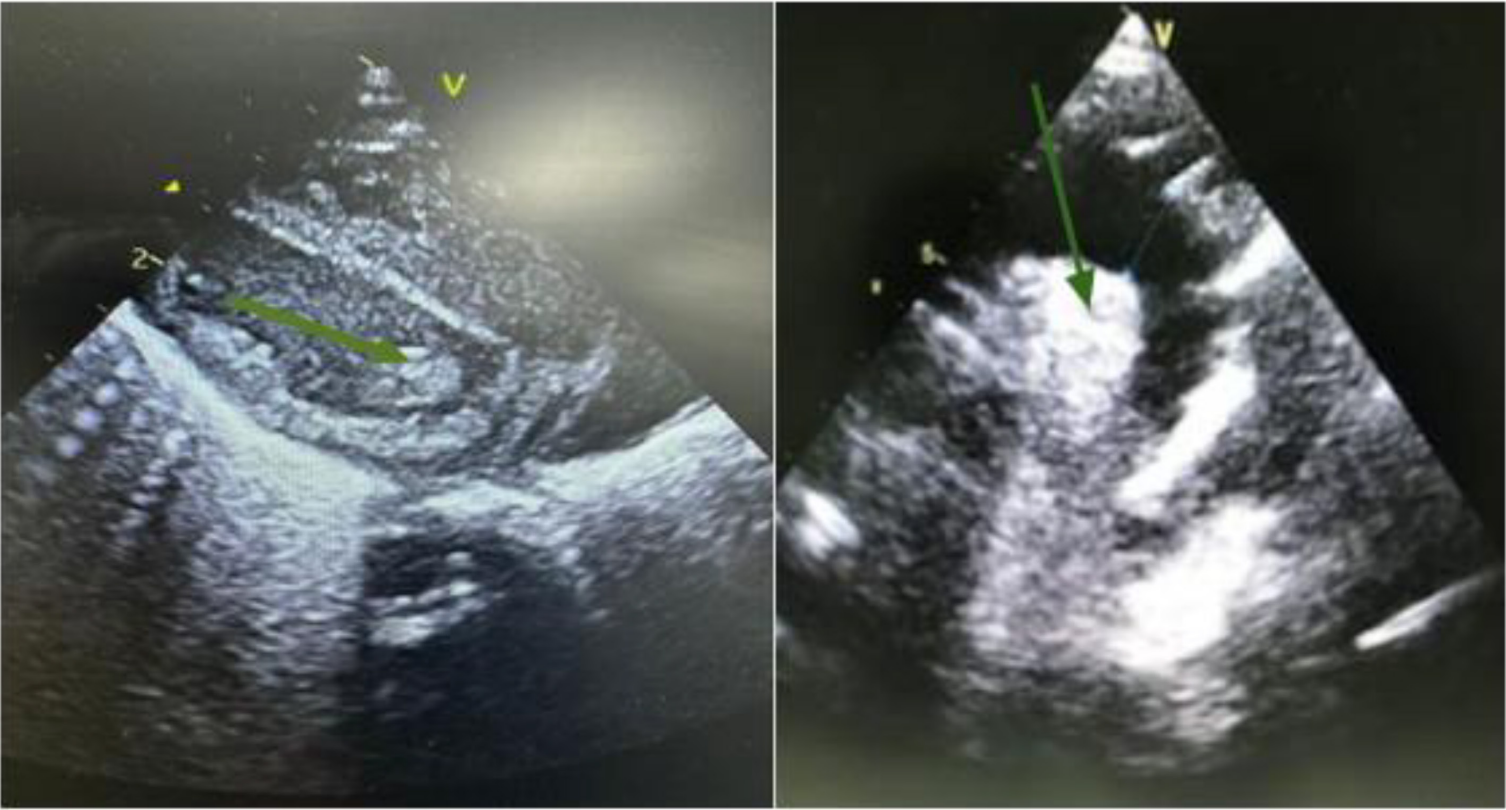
Echocardiographic image demonstrating a mitral valve vegetation (green arrow) in the setting of IE. The irregular, mobile mass is attached to the mitral valve leaflets, causing obstruction and regurgitation. This finding is characteristic of bacterial infection involving the heart valves.
